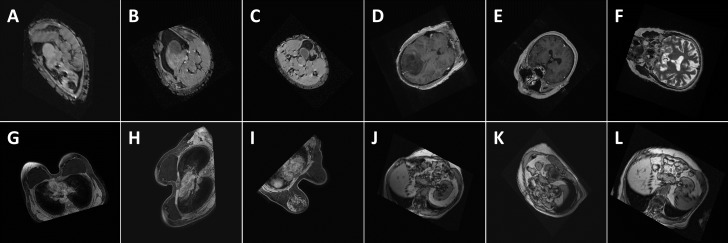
Performance (confidence level of classification) of the proposed method with respect to some random images: (A) Abdomen, 85.7%, (B) Abdomen, 92.3%, (C) Abdomen, 94.2%, (D) Head, 99.9%, (E) Head, 100%, (F) Head, 98.8%, (G) Breast, 100%, (H) Breast, 100%, (I) Breast, 100%, (J) Thorax, 98.9%, (K) Thorax, 98.9%, and (L) Thorax, 99.7%. The images show the views extracted using the proposed algorithm from 3D CT and MRI images in the test databases. Note that the differences in the images of the same class are caused by the simplicity of the segmentation method which influences the symmetry plane extraction.
